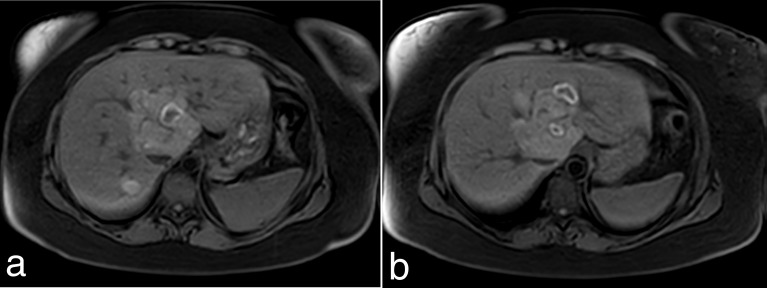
MRI September 2016. Consecutive slice pre-gadolinium *T*_1_ FATSAT through the liver demonstrates high signal rim around the high *T*_2_ SPAIR signal lesions.
